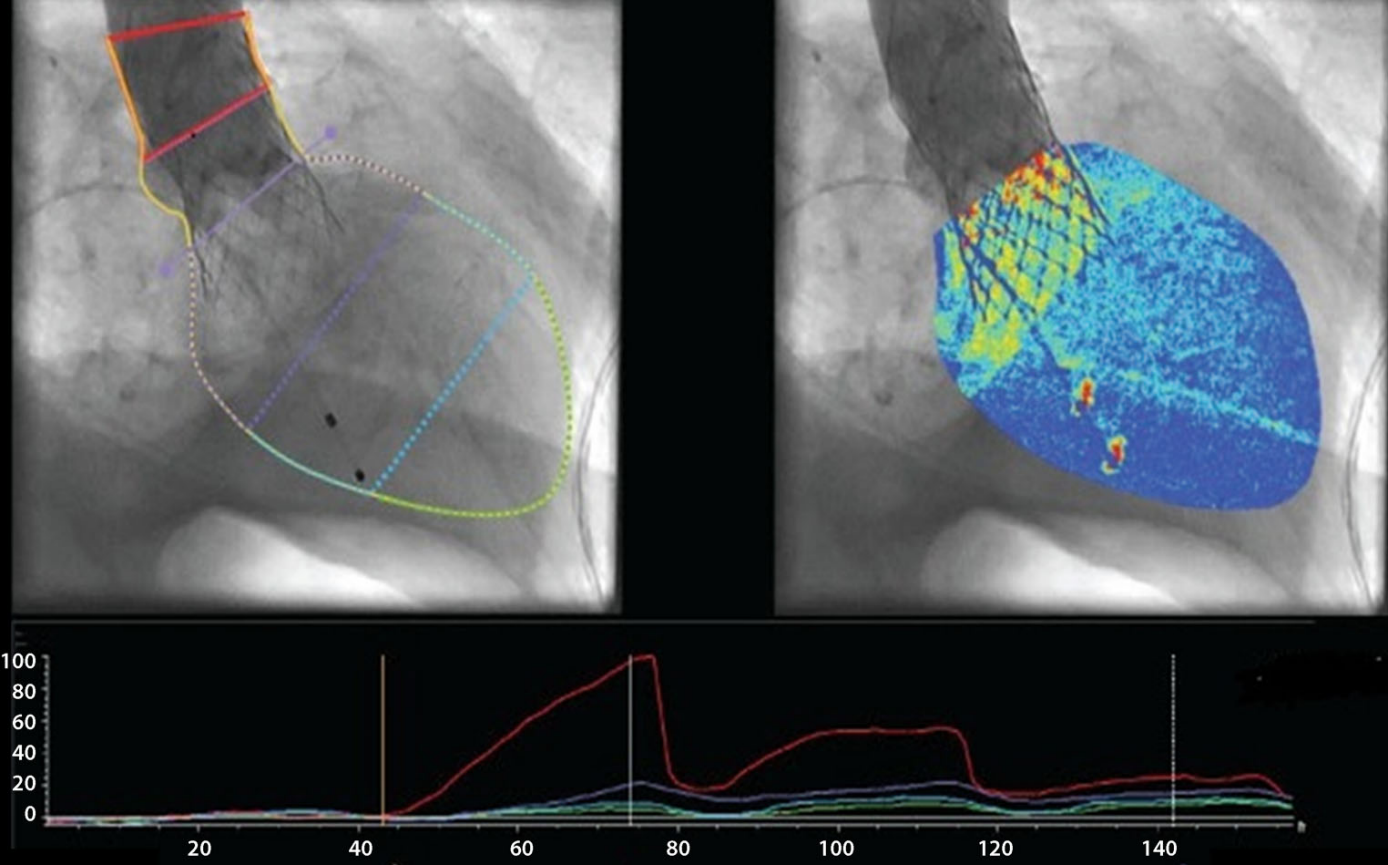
X-ray contrast densitometry with the special software (CAAS A‑Valve) after transcatheter aortic valve implantation
